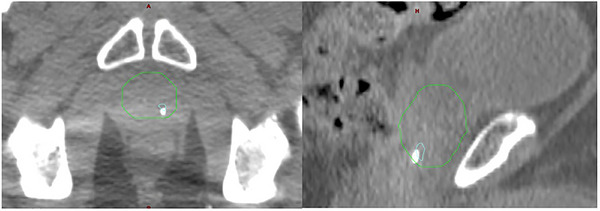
CBCT acquired during radiation treatment in the axial view (left) and sagittal view (right) from Varian's Offline Review, showing the fiducial marker residual error after the CBCT matching. Fiducial marker (blue contour); prostate contour (green).
